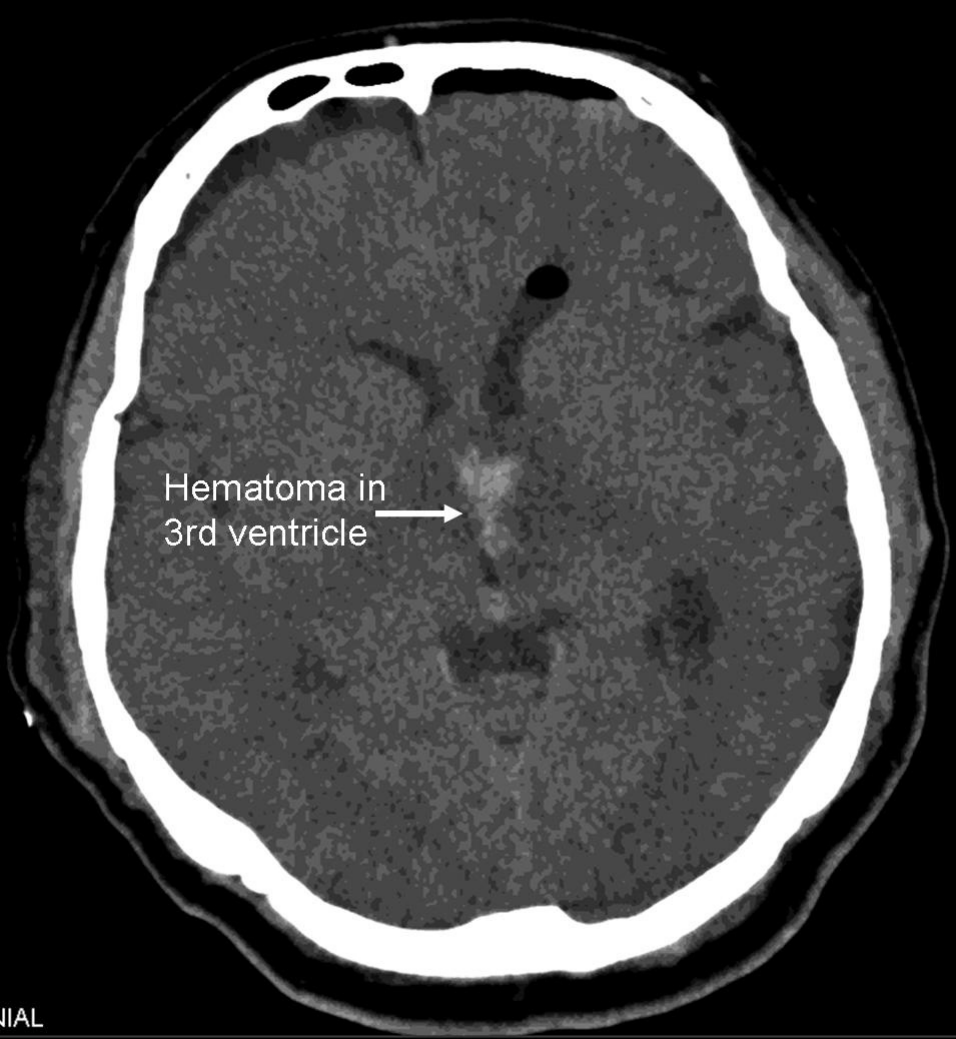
A cranial CT scan performed three days post-operatively showing a hematoma in the surgery field with no indications of hydrocephalus.
